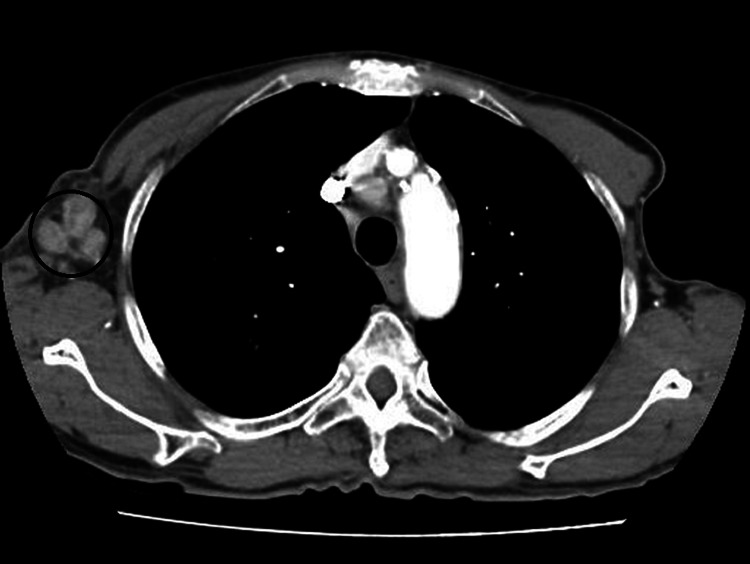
CT chest soft tissue window showing right axillary lymph nodes (marked with a black circle) on 06/12/2017
